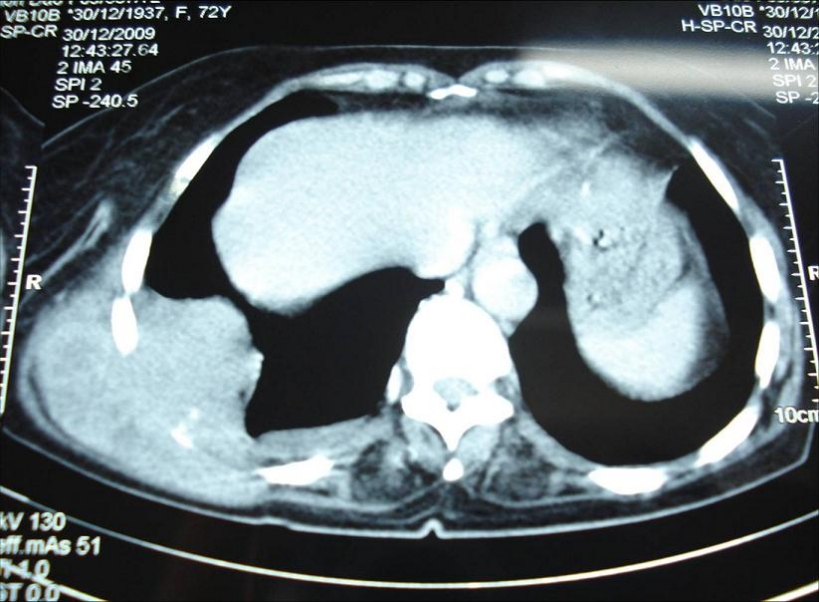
Computed tomography scan of the chest:Soft tissue mass lesion in posterior chest wall with thorax extension accompanied by pleural thickening
